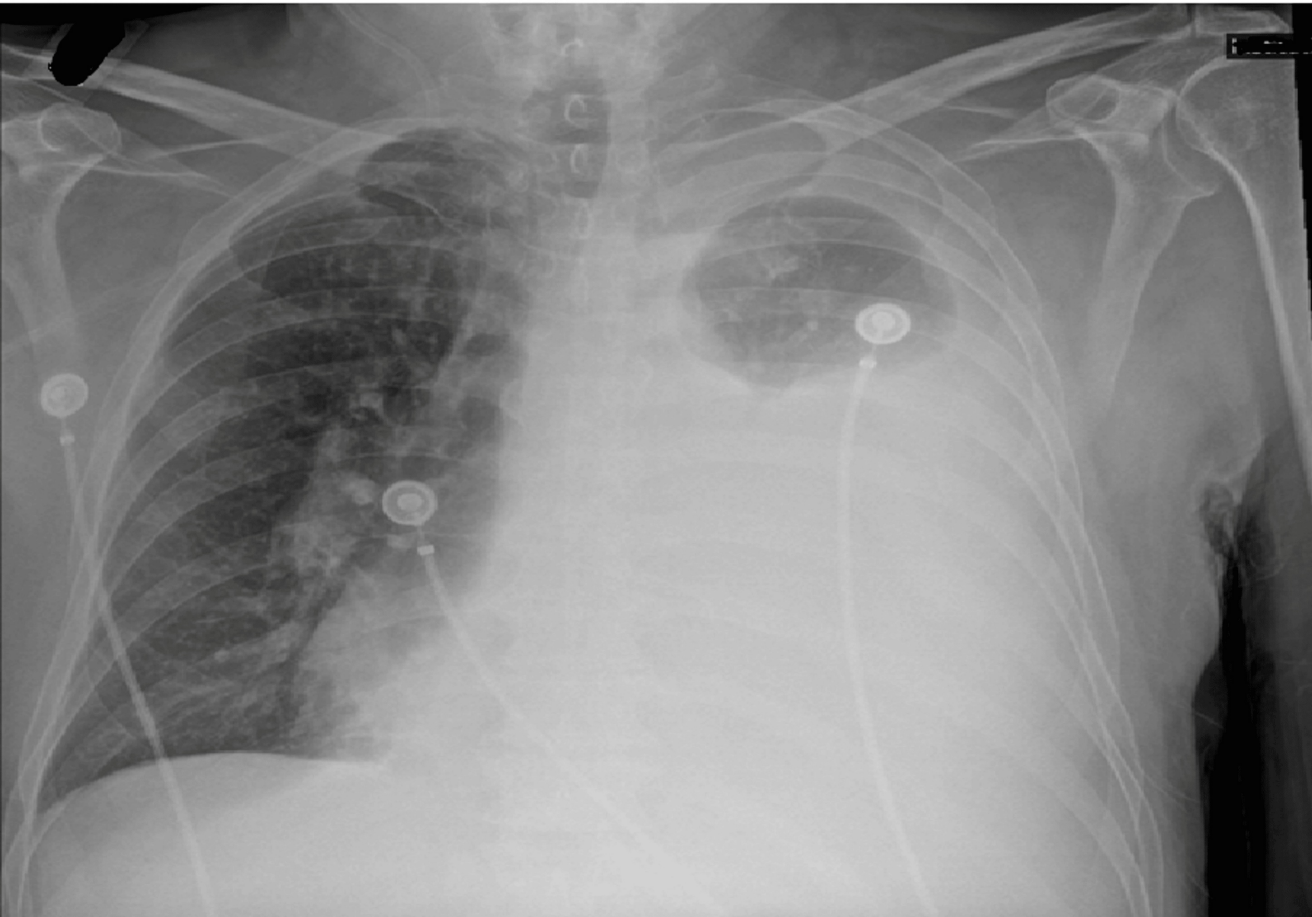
Chest X-ray Large left pleural effusion with minimal aeration of the left upper lobe.
